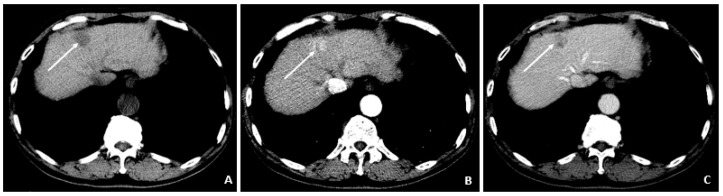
Triphasic CT at the time of HCC diagnosis (March 2009). (A) The precontrast image shows a hypodense lesion (arrow) at the site of the punctate hyperenhancing lesion shown in Figure 4. (B) The corresponding arterial-phase postcontrast image reveals hyperenhancement (arrow). (C) The portal-phase postcontrast image shows washout (arrow), and all features are typical of HCC and confirm the diagnosis in the setting of chronic HBV.
